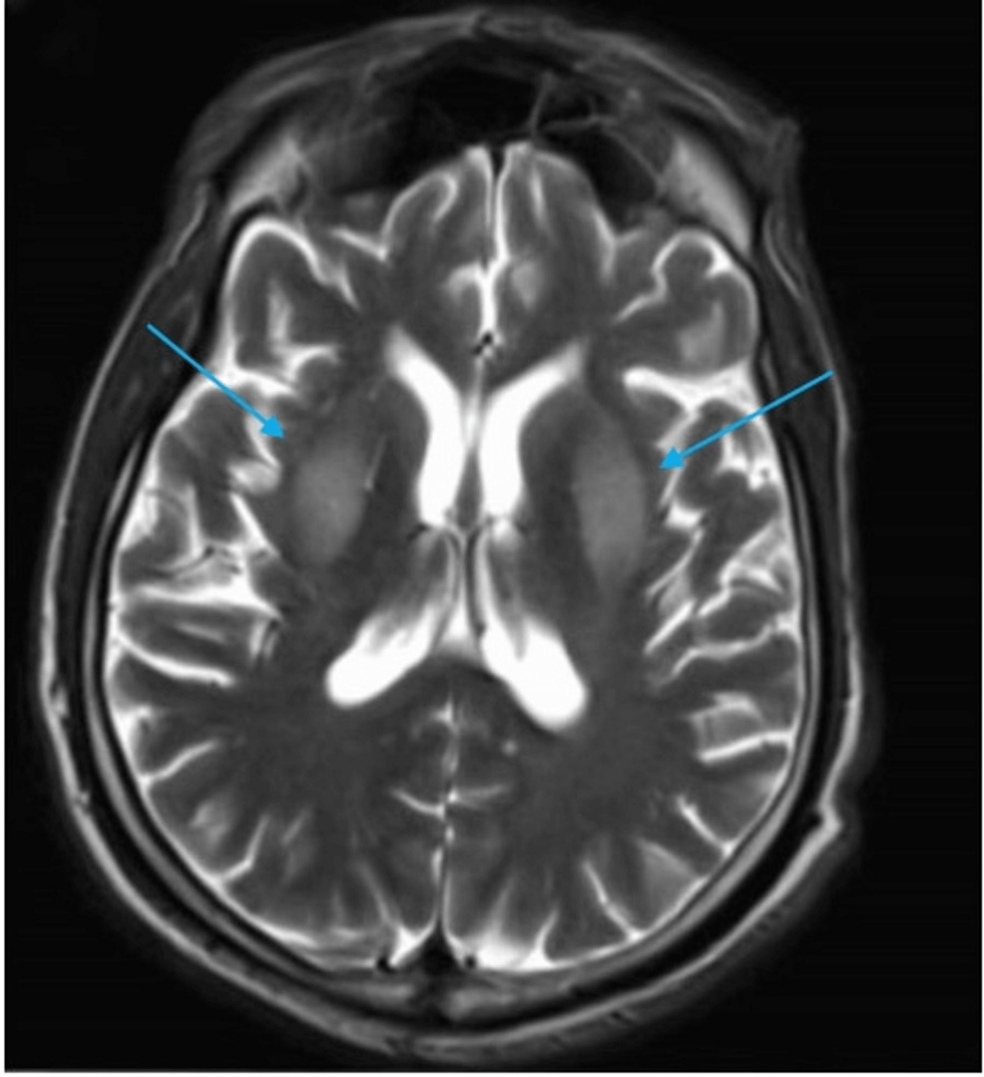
MRI brain: Day-60 axial T2-weighted image shows recurrence of symmetric hyperintense lesions in bilateral basal ganglia (blue arrows)
